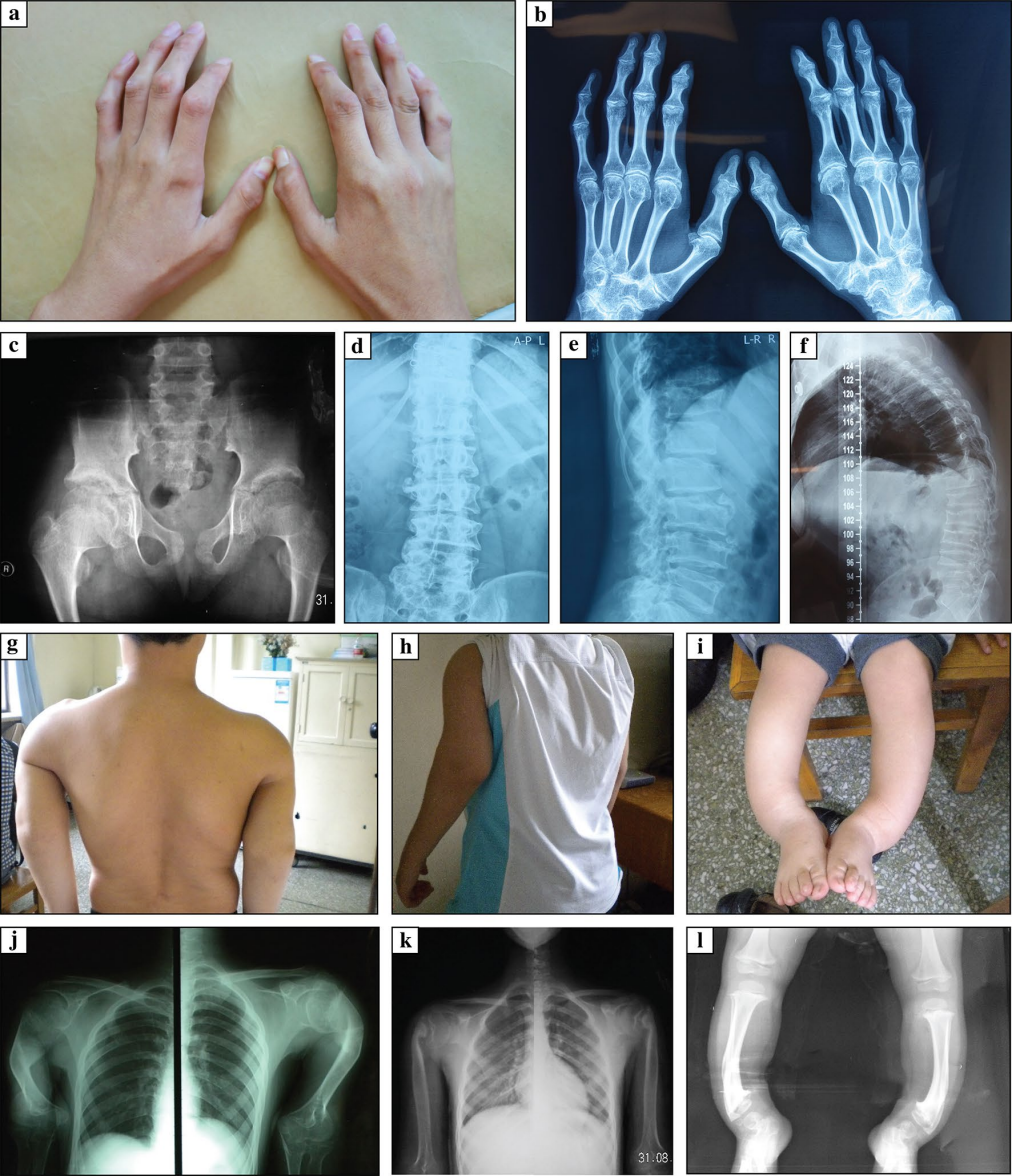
Clinical and Imaging findings of progressive pseudorheumatoid dysplasia (PPRD). Typical imaging findings of PPRD (a–f) and imaging findings of PPRD cases with atypical severe long bone involvement (g–l). a, b Flexion deformity and claw shape of bilateral hands. X-ray showed osteoporosis of bilateral hands, epiphyseal enlargement of the metacarpophalangeal, proximal and distal interphalangeal joints, narrowing of the metacarpophalangeal joint spaces, irregularity of articular surfaces, and joint deformities (case 1); c widening of the pubic symphysis and bilateral sacroiliac joint spaces. Osteoporosis and degeneration of the bilteral hip joints, narrowed hip joints spaces, and ill-defined articular surfaces can be observed. Cystic changes were under the articular surfaces of bilateral femoral heads. The femoral necks were also shortened (case 2); d–f scoliosis or kyphosis of the spine (d and e from case 1; f from case 3). The vertebral bodies were flattened. There was a lack of regularity on the superior and inferior surfaces; g, j shortening and flexion deformities of the bilateral humeri with metaphyseal or epiphyseal enlargement of the upper and lower ends. The elbow joints were enlarged with narrowed joint spaces. There might be a rotational deformity of the humerus on the right shoulder (case 4); h, k enlargement of the humeral head. The humeral neck was narrowed. There might be a flexion deformity (case 1); i, l varus deformities of the bilateral lower extremities. The tibia and fibula on the bilateral sides were symmetrically curved. There were metaphyseal enlargements of the knees and ankles, and a widening of the joint spaces (case 5, the younger brother of case 1)
